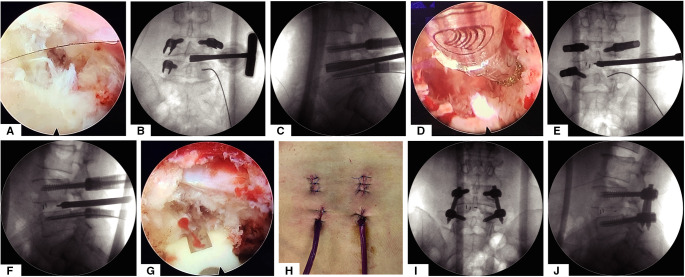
The whole process of decompression and intervertebral disc treatment and implantation of intervertebral fusion cage under the visual channel. (A) Endoscope visual field assisted by visual working channel after articular process osteotomy and decompression of the ligamentum flavum. (B,C) The AP and lateral view of x-ray for discectomy by various paddle distractor. (D) Endoscopic view of endplate preparation by using turnable burrs. (E,F) Implant an intervertebral fusion cage under the guidance of AP and lateral view of x-ray. (G) Endoscopic view after implanting the intervertebral fusion cage. (H) General view of the postoperative incision. (I,J) AP and lateral view after surgery.
